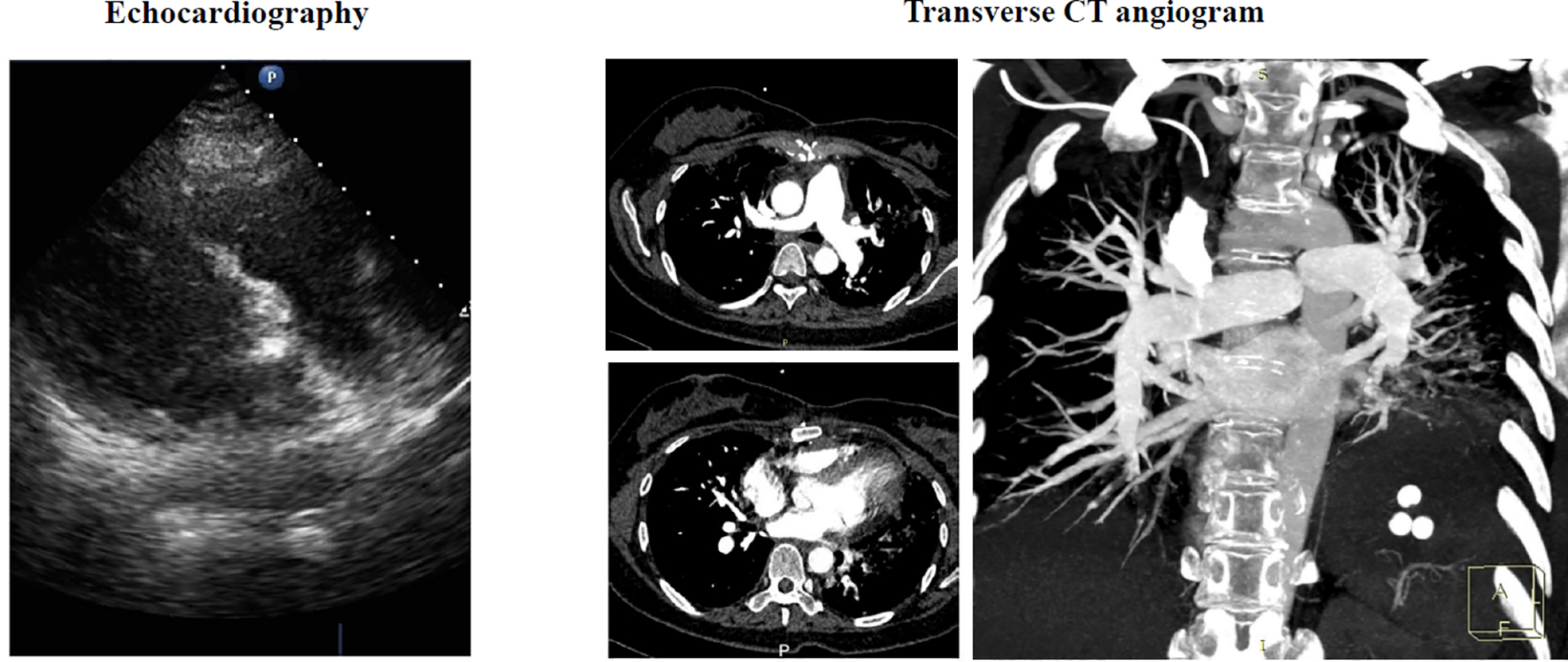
Postoperative echocardiography and transverse CT angiogram showed that most tumor tissue of both lungs has been resected with a little residual occlusion.
